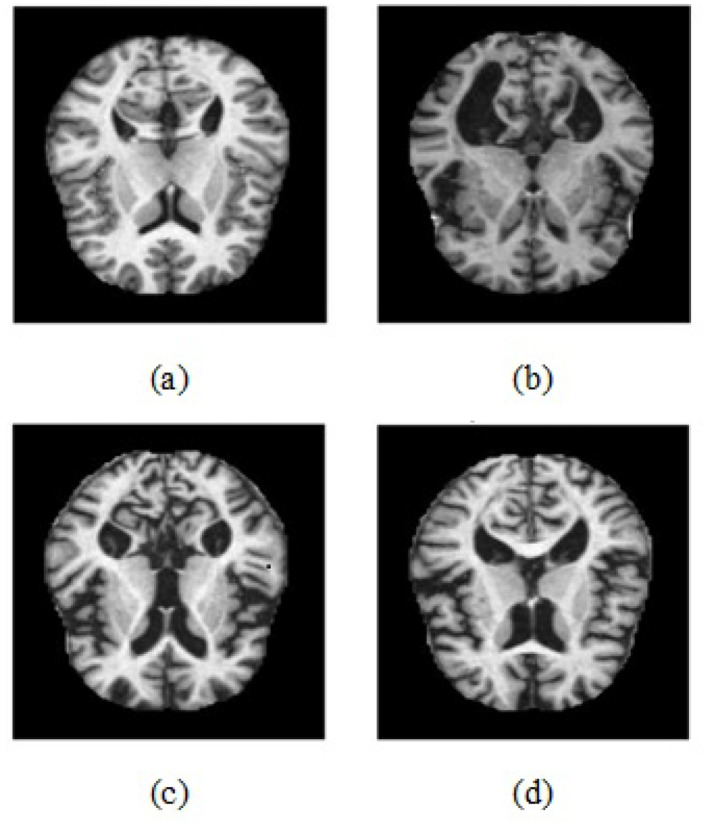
MRI images of AD: (a) non-demented (ND), (b) mildly demented (MID), (c) moderately demented (MOD), (d) very mildly demented (VMD).
